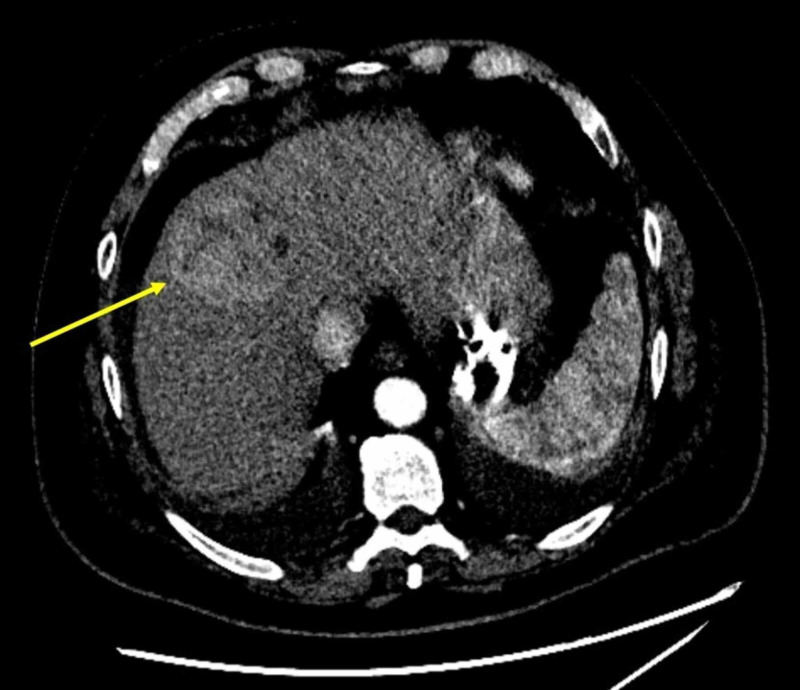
Arterial phase of triple phase CT abdomen The yellow arrow points at hepatocellular carcinoma.
